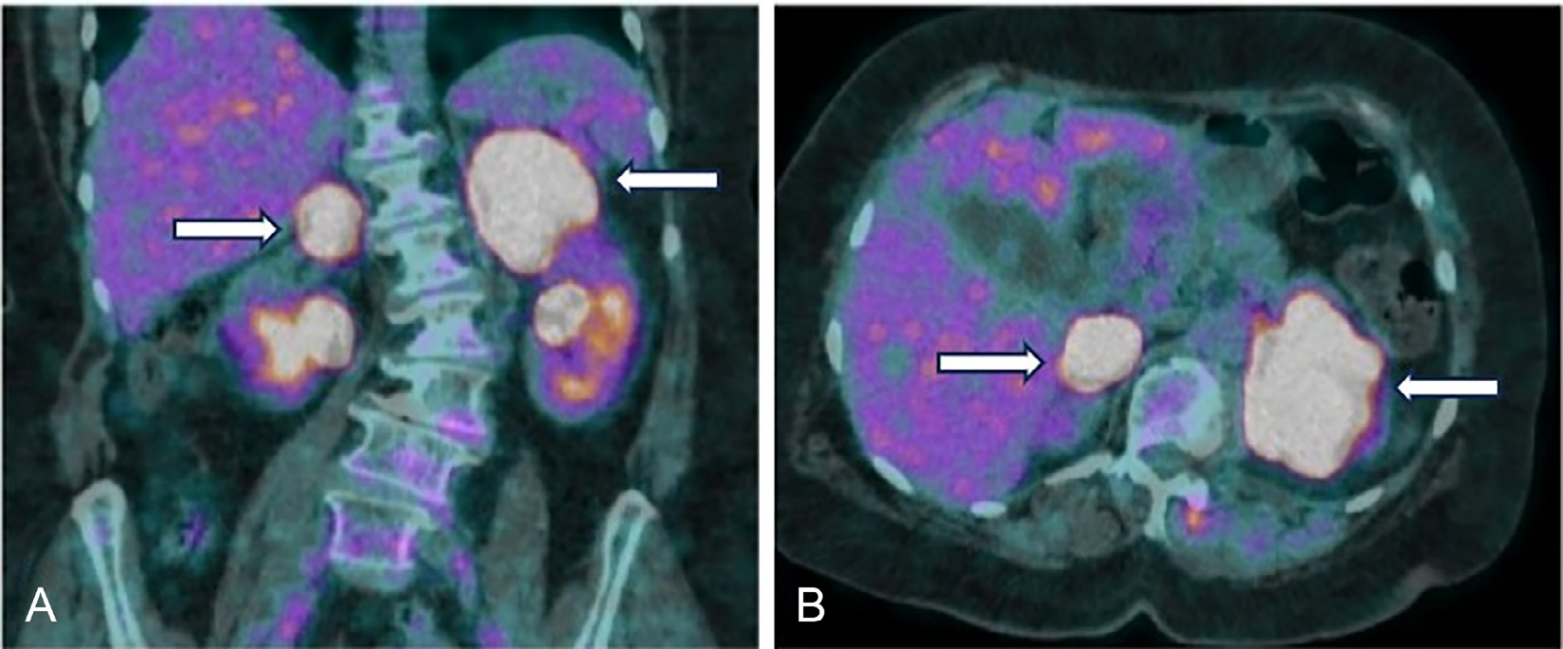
Axial and coronal fusion images from end-of-treatment PET-CT after 6 cycles of CHOP and 2 cycles of high-dose methotrexate. Imaging demonstrates interval progression in size and metabolic activity of bilateral adrenal masses, representing metabolically progressive disease and treatment failure.
